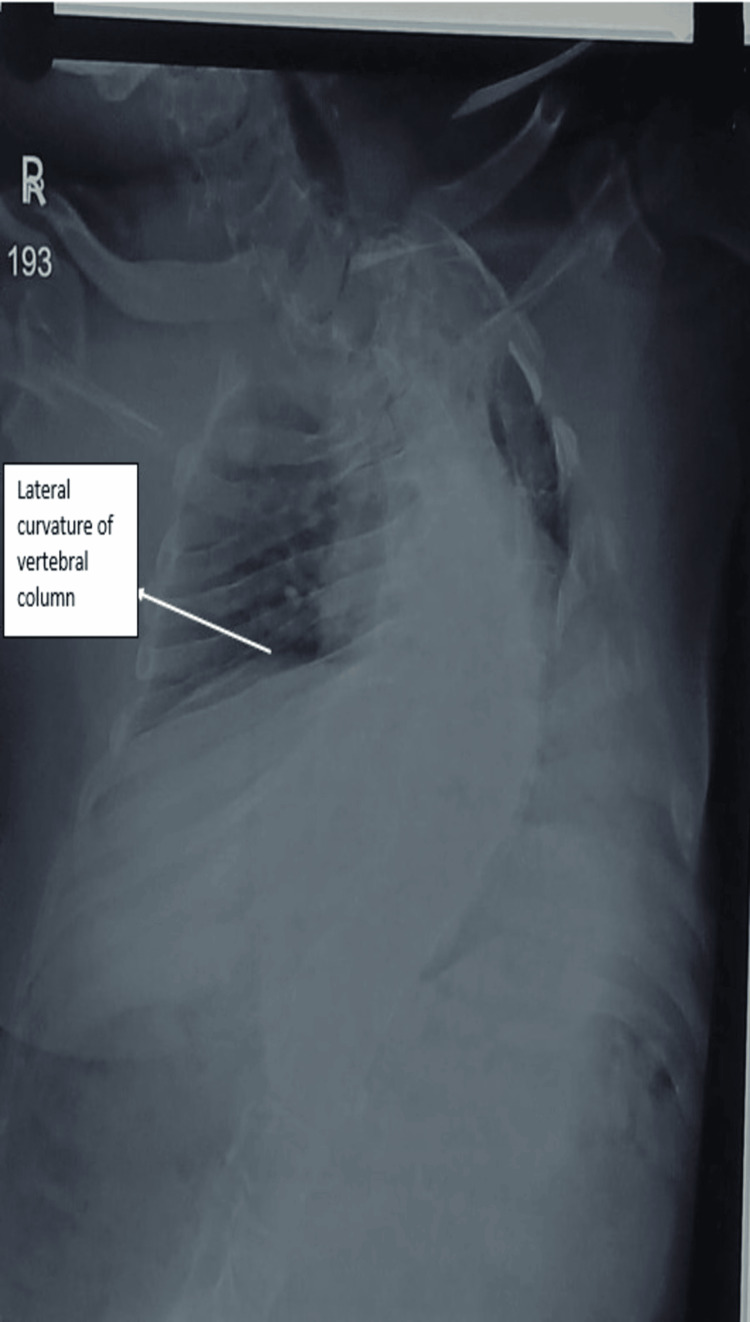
Chest radiograph showing abnormal curvature of the thoracic spine consistent with kyphoscoliosis. The white arrow indicates the lateral curvature of the vertebral column. This spinal deformity contributed to altered body alignment and indirectly influenced pelvic orientation and ureteral course during ureteroscopic intervention.
